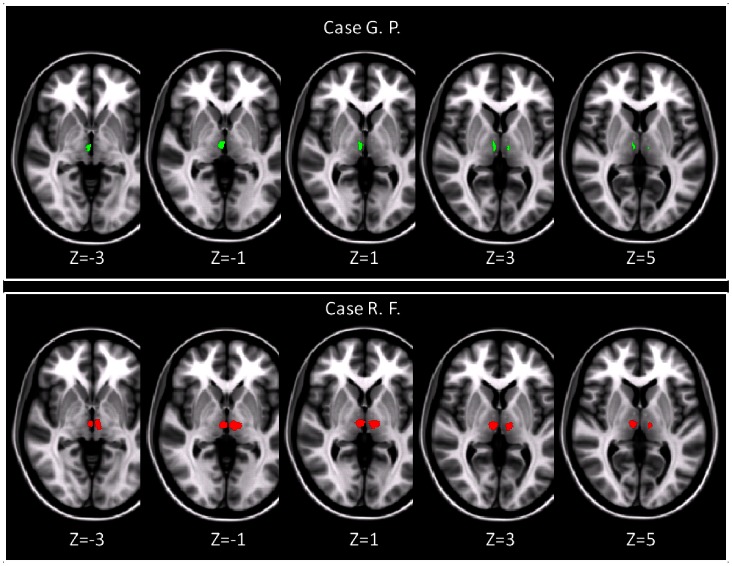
Location of the thalamic lesions after warping to MNI space. Lesion masks are shown for both, G. P. (A) and R- F. (B). The resliced lesion masks are overlaid onto the FSL T1-weighted template in standard space. The labels indicate the slice coordinate in MNI space.
